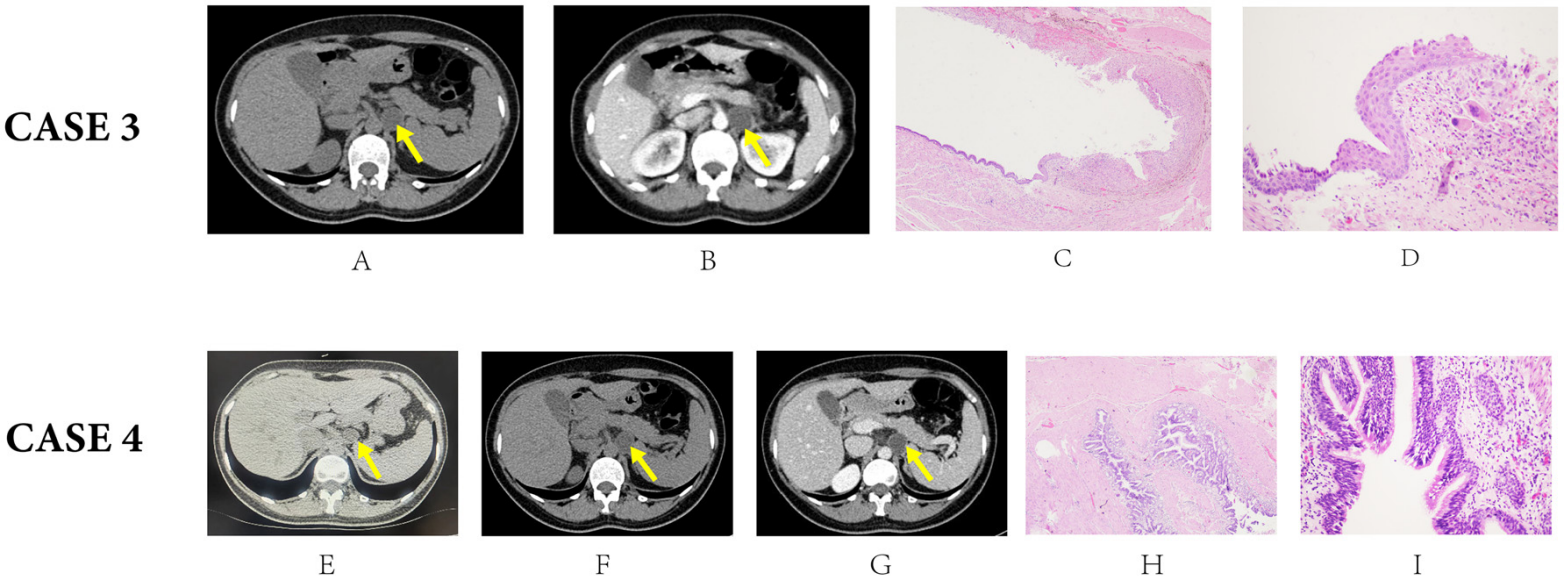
CT and pathological manifestations of Case 3 (A-D) and Case 4 (E-I). The yellow arrow shows the location of the cyst: (Case 3: (A) non-contrast enhanced stage: A 31HU 2.7*2.2cm soft-tissue nodule in the left adrenal area; (B) contrast enhanced stage: insignificant enhancement after contrast agent injection; (C): low-power magnification [40×]: The capsule wall was surrounded with smooth muscle and fibrous connective tissue. (D): high-power magnification [100×]: Migration area of ciliated columnar epithelium, squamous epithelium, and foam cell surrounded by deposition of hemosiderin and vasodilation congestion. Case 4: Image (E) was taken two years ago when the mass was first detected. Image (F, G) were taken in our outpatient clinic at the time of admission. The yellow arrow shows the location of the cyst: (E) The CT image showed a 2.0*2.1 cm ovoid low-density mass. (F) non-contrast enhanced stage: A low-density mass in the left adrenal area with a diameter of 2.5 cm; (G) contrast enhanced stage: Insignificant enhancement after contrast agent injection. H: low-power magnification [40×]: Cystic structure was surrounded by smooth muscle with ciliated columnar epithelium inside the cyst wall. I: high-power magnification [100×]: Infiltration of mucinous glands and lymphocytes).
